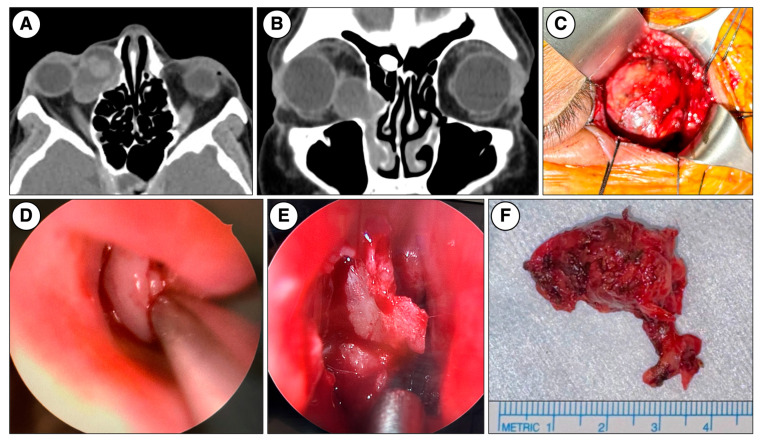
Combined external and endoscopic approach for en bloc resection of a non-keratinizing squamous cell carcinoma of the lacrimal drainage system in a 68-year-old woman with prior dacryocystorhinostomy and multiple resections for recurrent transitional-cell papillomas. (A,B) CT revealed a large, circumscribed lesion with heterogeneous contents, extending inferomedially into the right orbit and nasal cavity through the previous rhinostomy, along with enlargement of the right nasolacrimal duct. (C) External exposure of the tumor. (D) Endoscopic view of the inferior meatus showing tumor involvement. (E) A prelacrimal recess approach with removal of the medial bony wall of the nasolacrimal duct facilitated en bloc resection of the tumor (F).
